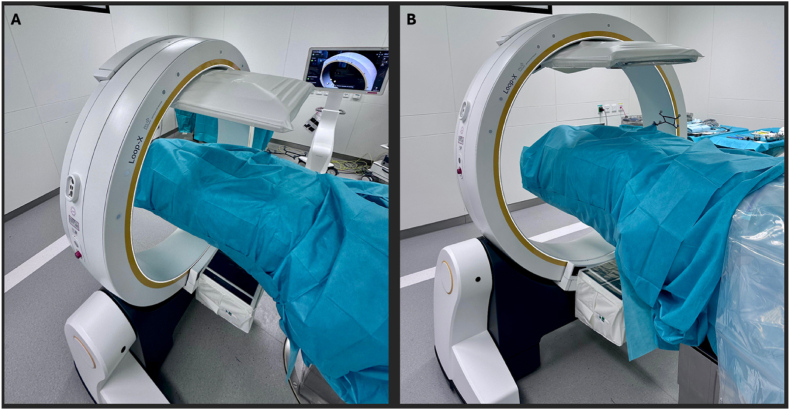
Loop-X positioning and intraoperative 3D acquisition. (A) The ring-shaped Loop-X gantry positioned around the prone patient prior to scanning. (B) Intraoperative cone-beam CT acquisition of the lumbar spine.
